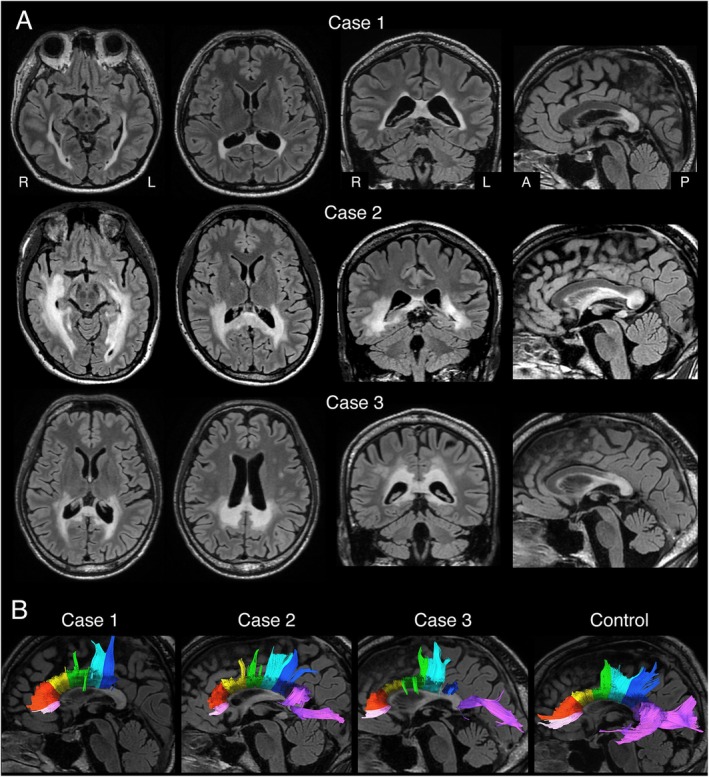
Neuroimaging findings. (A) Brain magnetic resonance imaging (MRI) showed fluid‐attenuated inversion recovery (FLAIR) hyperintense lesions in the bilateral occipitotemporal white matter and splenium of the corpus callosum in Cases 1 and 2, and in the bilateral parieto‐occipital white matter, splenium of the corpus callosum, and retrosplenial white matter and fornix in Case 3. Loes scores were 7, 12.5 and 12 for Cases 1, 2, and 3, respectively. (B) Sagittal views of three‐dimensional reconstructions of callosal fibers overlaid on midsagittal images in Cases 1–3 and a control subject with adrenomyeloneuropathy. Fibers were classified according to Witelson's scheme and color‐coded as follows: Pink, rostrum; red, genu; yellow, rostral body; green, anterior midbody; light blue, posterior midbody; dark blue, isthmus; and purple, splenium. Compared with the control subject, all three cases showed reduced splenial fibers, which was most severe in Case 1, in whom splenial fibers were not reconstructed. Case 3 also showed reduced isthmus fibers.
